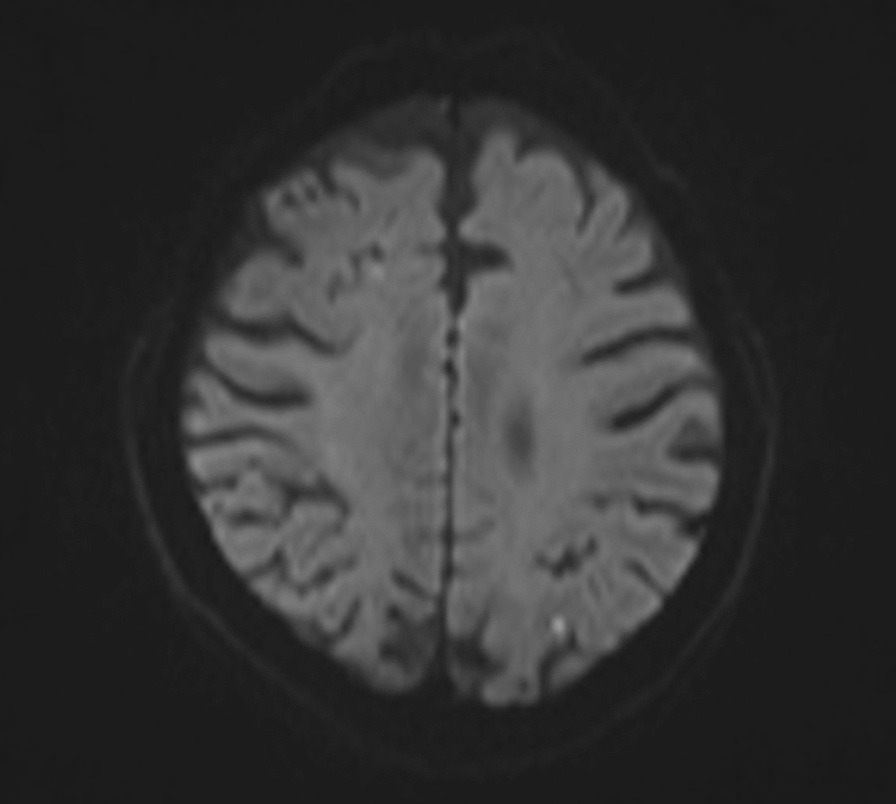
MRI noted only a small old infarction in the left parietal lobe
